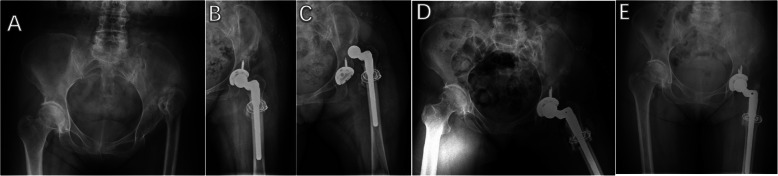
Radiographs of a 48-year-old female patient with high hip dislocation secondary to childhood pyogenic infection that had a quiescent period of 40 years between infection and left THA. a Preoperative AP pelvic radiograph. b Immediately postoperative AP hip radiograph. c Dislocation of the left hip 6 days postoperatively. d Postoperative dislocation treated with closed reduction. e Postoperative AP pelvic radiographs 4 years after THA
